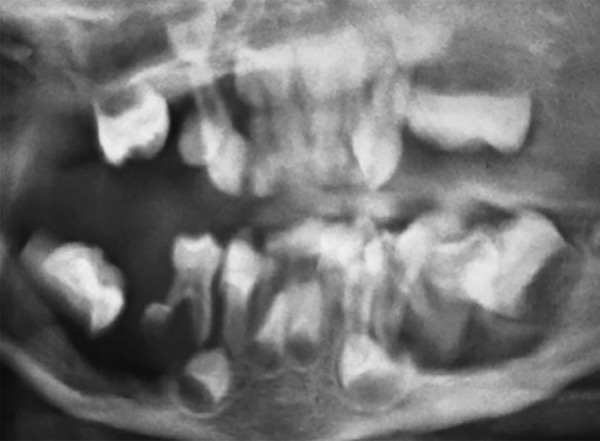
OPG X-ray revealed multiple radiolucent lesions and foating teeth
